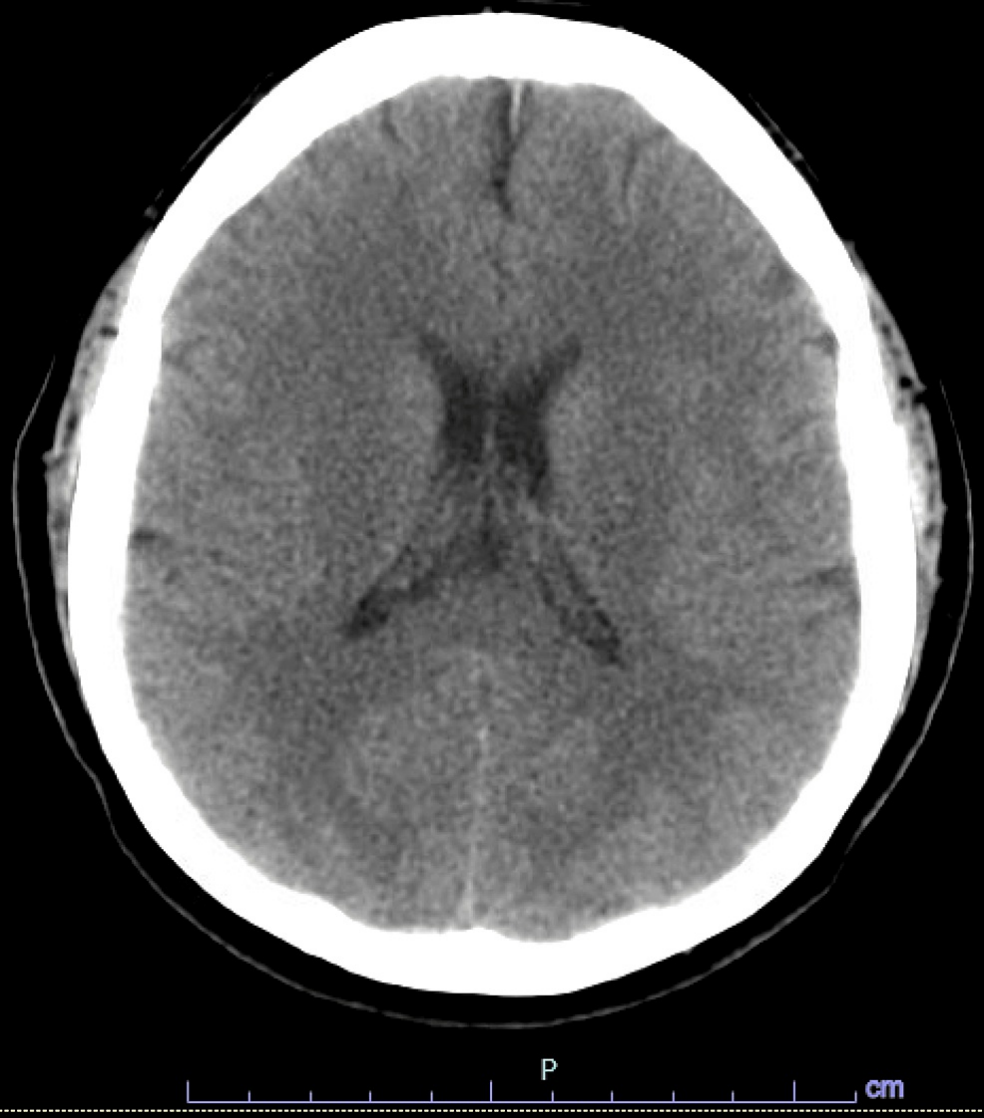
CT of the head and brain, axial view obtained on admission without evidence of intracranial hemorrhage, intracranial infarction, or traumatic brain injury. No mass effect, edema, midline shift, or herniation.
